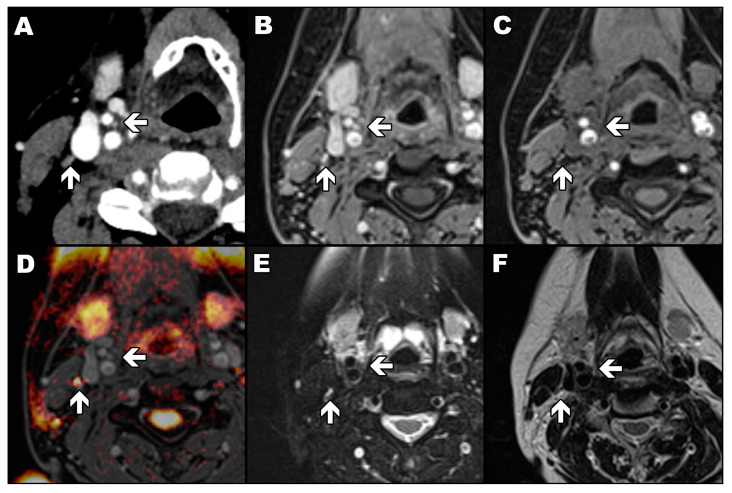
Signal characteristics of the carotid body and lymphatic node. Arrows mark the carotid body (←) and lymph node (↑). Axial contrast-enhanced computed tomography image of the patient with marked carotid body and lymph node (A); VIBE sequence in T1-weighted image with Dixon technique in the water-only image with (B) and without (C) contrast agent enhancement; diffusion-weighted image with *b* value of 1000 s/mm^2^ automatically fused with contrasted-enhanced VIBE sequence in T1-weighted image with Dixon technique in the water-only image (D); turbo spin-echo sequence in T2-weighted image with (E) and without (F) fat saturation.
